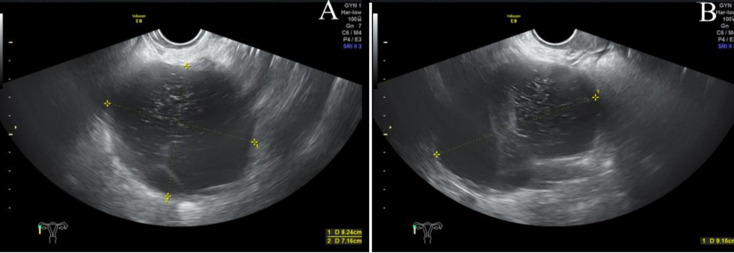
transvaginal 3D Ultrasound Imaging of the uterine adnexa; A) a well-defined, anechoic cystic mass (82x72 mm in transverse and anteroposterior dimensions) in the right adnexa; B) the same right adnexal mass, measuring 92 mm in length, with internal septations and fine echogenic spots
